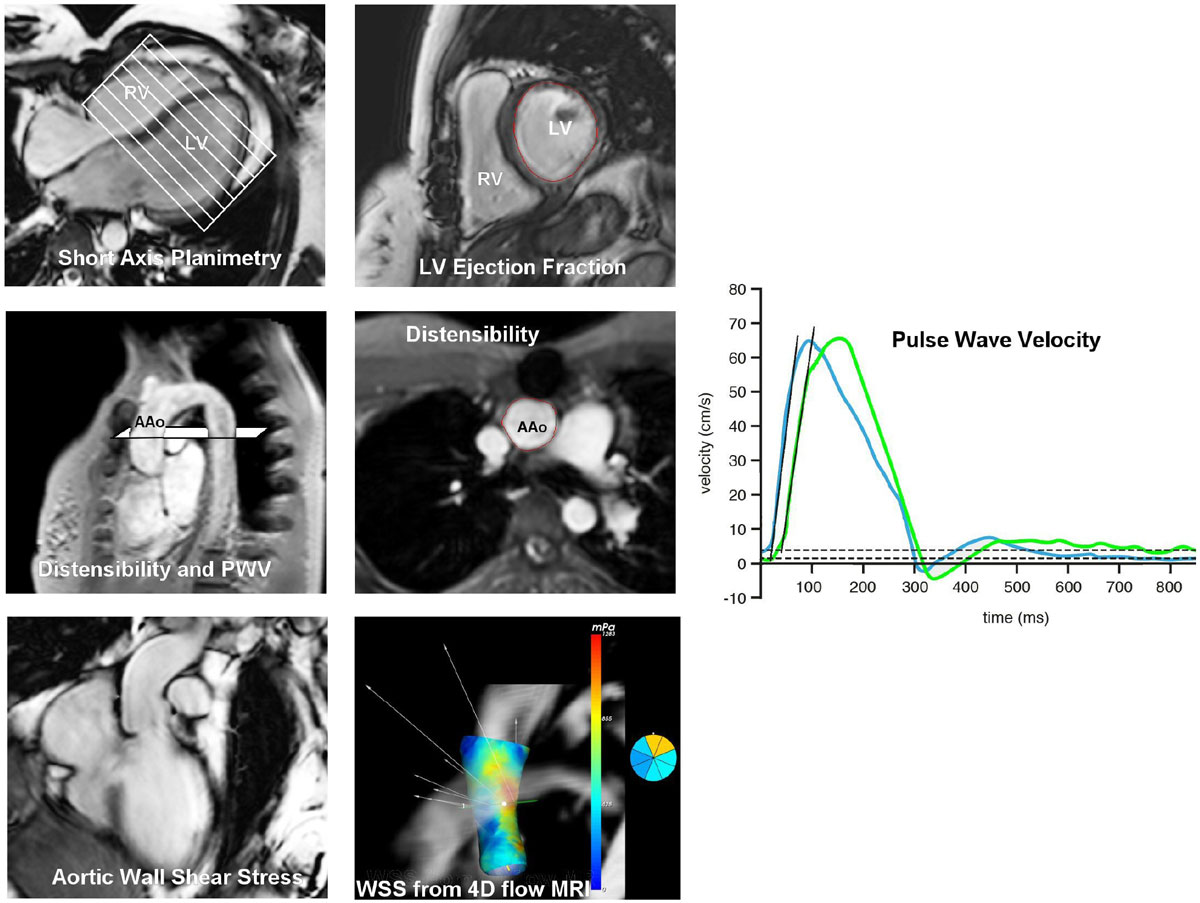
CMR evaluation: LV short-axis evaluation to calculate LV ejection fraction (top row), time-resolved 2D velocity encoded MRI to determine Pulse Wave Velocity over the aortic arch and Distensibility of the ascending aorta (AAo) (middle row), 4D flow MRI to determine the wall shear stress in the ascending aorta (bottom row).
